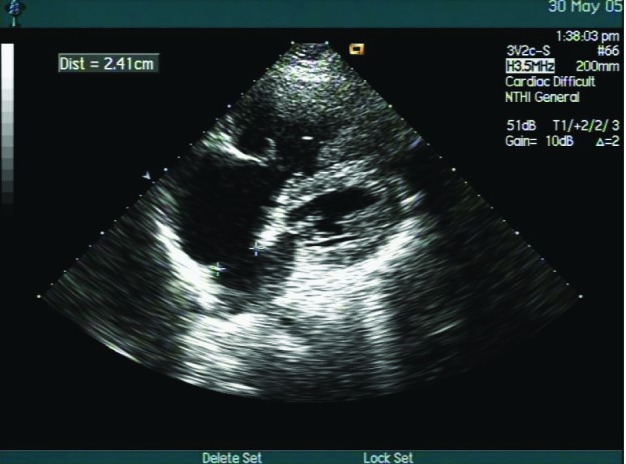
Ultrasound cardiogram showing the atrial septal defect.
